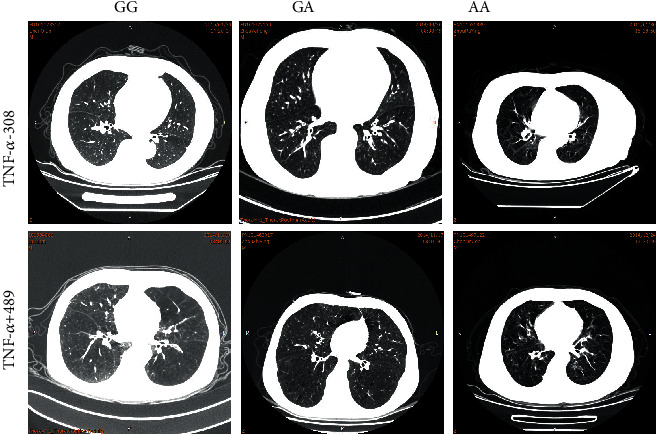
Differences among AECOPD patients with different genotypes on inspiratory-expiratory high-resolution computerized tomography (HRCT) imaging.
